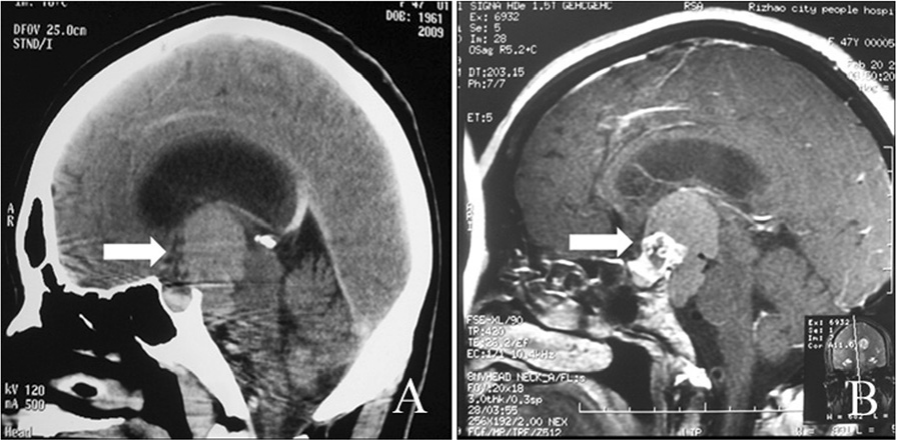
CT and MRI scans 11 months after the first operation. (A) Sagittal CT showing the isodensity in the suprasellar area and the prepontine cistern (arrow). (B) MRI showing a partial contrasting mass in the suprasellar area (arrow).
